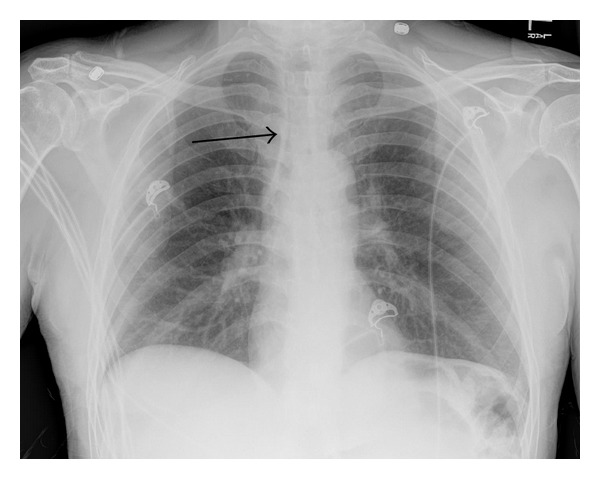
Anterior-posterior chest X-ray demonstrating tracheal wall thickening (arrow) as the result of alkaline ingestion.
